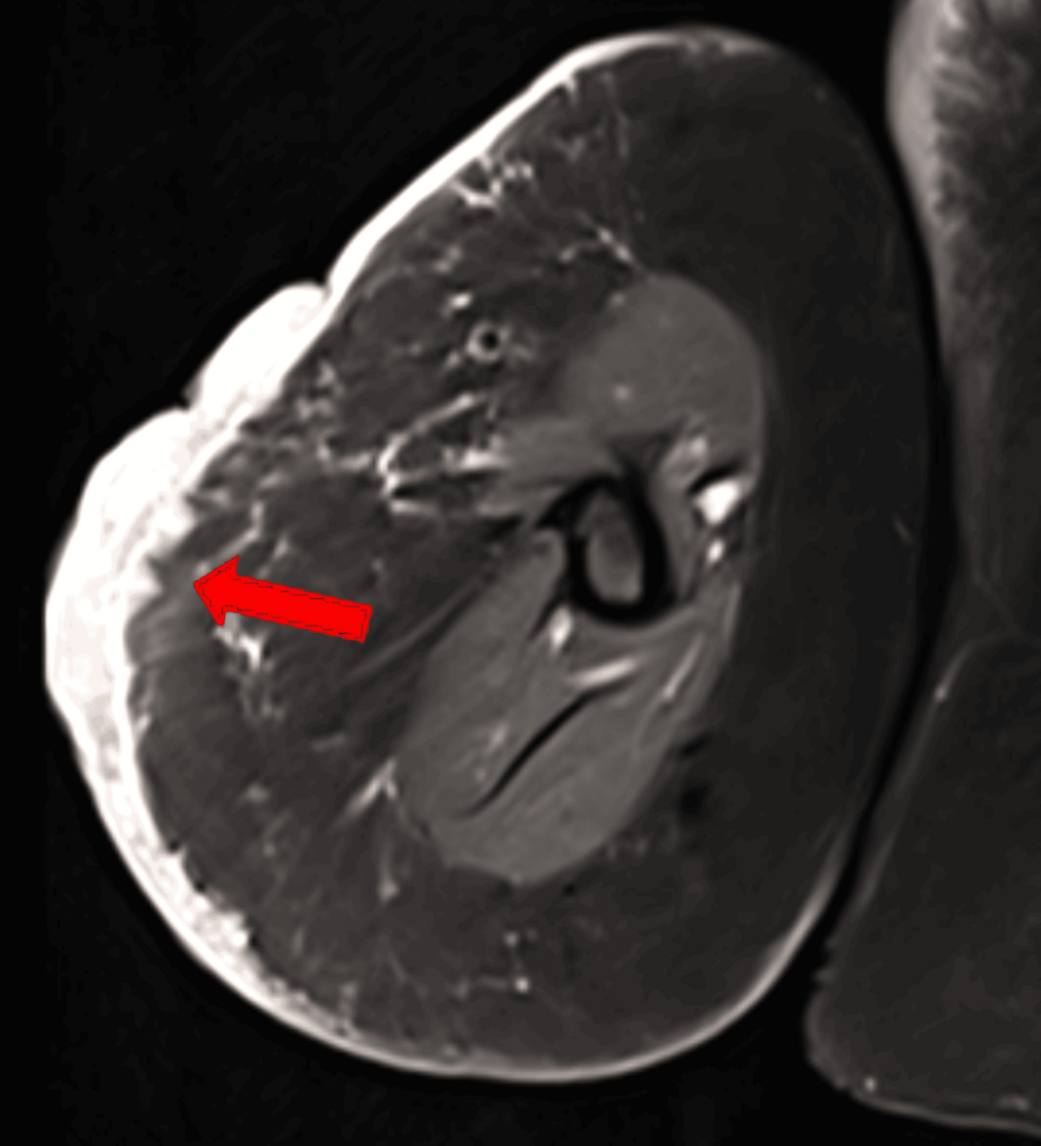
MRI showing an ill-defined lesion in lateral aspect of midarm in cutaneous and subcutaneous plane (arrow) MRI, Magnetic resonance imaging
